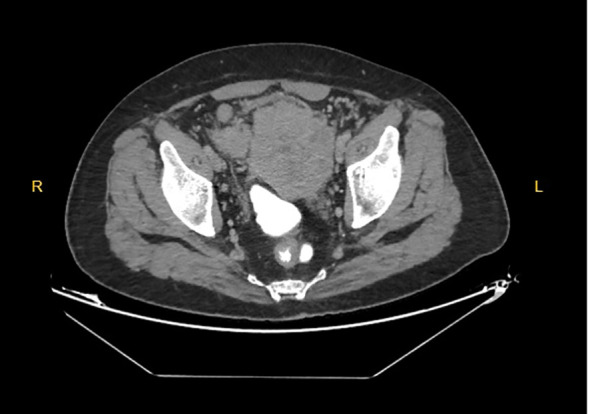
Contrast-enhanced CT image showing nodular thickening and suspected focal perforation of the sigmoid colon.
